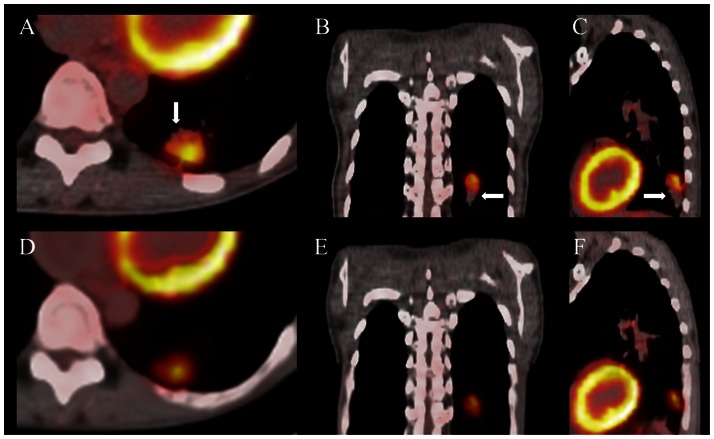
Represents the PET/CT fusion for tumor contour delineation. The arrows indicate the mismatch observed in PET_HCT_/HCT fusion between PET_HCT_ and HCT, showing the (A) transverse (B) coronal and (C) sagittal view. The image fusion with PET_IACT_ and IACT is seen in the (D) transverse (E) coronal and (F) sagittal view.
